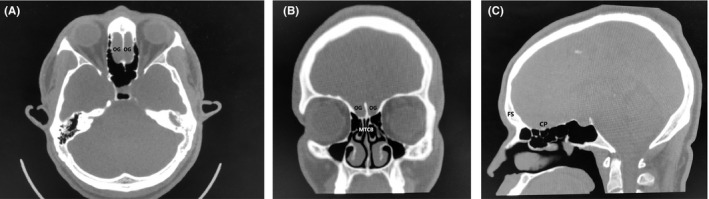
A, Axial CT view of patient with ONSD showing absent olfactory grooves (OG). B, Coronal CT view of patient with ONSD showing absent olfactory grooves (OG) and incidental middle turbinate concha bullosa (MTCB). C, Sagittal CT view of patient with ONSD showing underdeveloped cribriform plate (CP) with absent olfactory grooves and absent fenestrations for olfactory filaments and absent frontal sinuses (FS)
